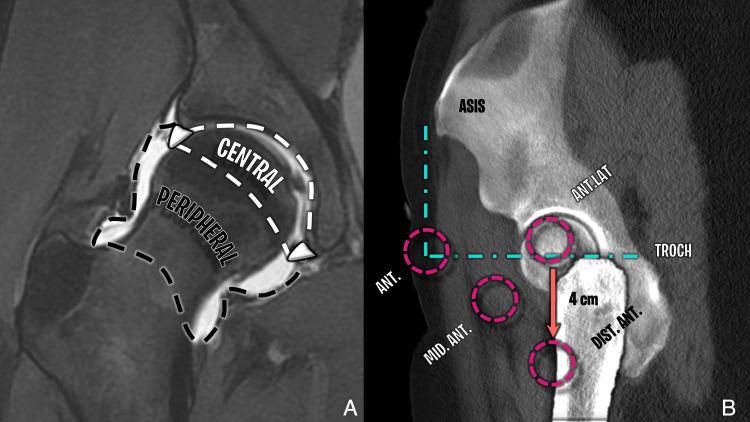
Hip joint compartments and arthroscopic portals (A) Coronal T1-weighted fat-saturated arthrogram showing central (white dotted line) and peripheral (black dotted line) compartments. Articular surfaces are only visible with traction. (B) Reformatted sagittal CT with portal overlays showing common outside-in arthroscopic portals. Image Credit: Juan Pablo Muñoz, corresponding author ASIS: anterior superior iliac spine; ANT: anterior portal; ANT.LAT: anterolateral portal; MID.ANT: mid-anterior portal; DIST.ANT: distal anterior portal
